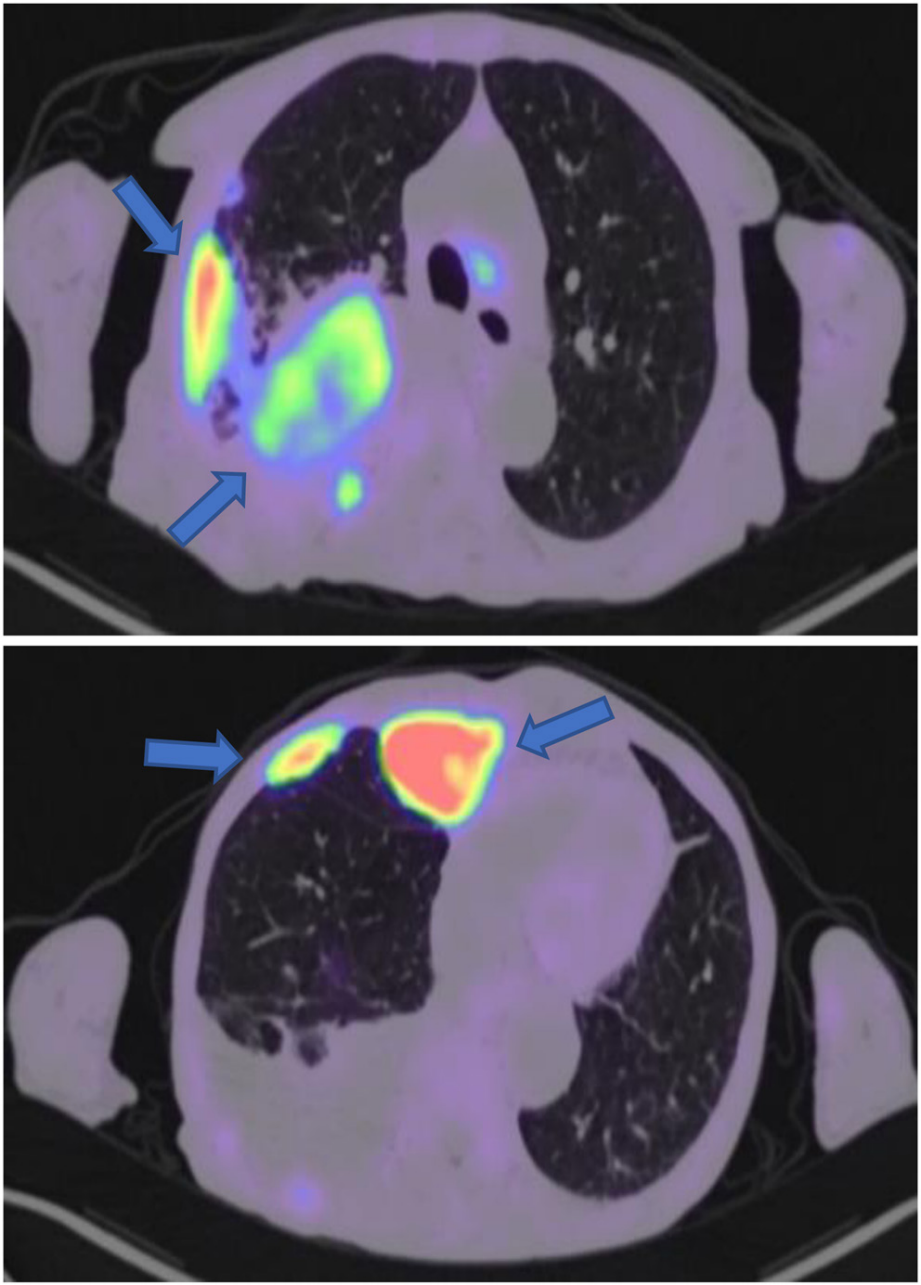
^68^Ga-DOTATATE PET/CT scan with arrows pointing to the upper lobe primary, pleural and nodal disease.
